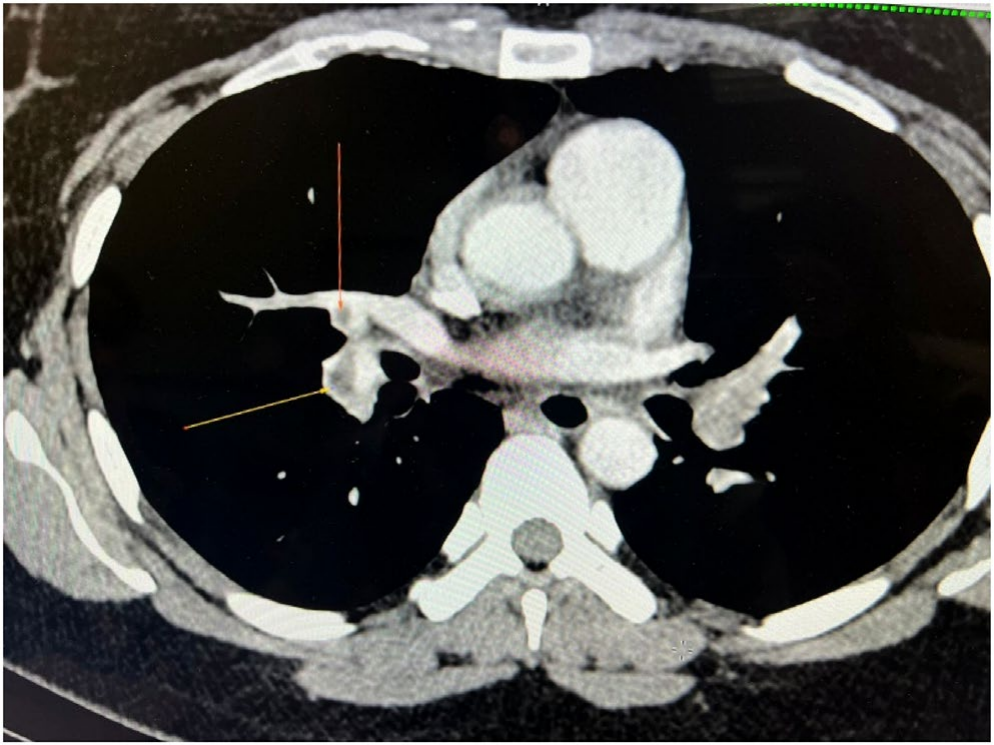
Shows a CT angiography image of the pulmonary arteries revealing an occlusion in the right main pulmonary artery.
